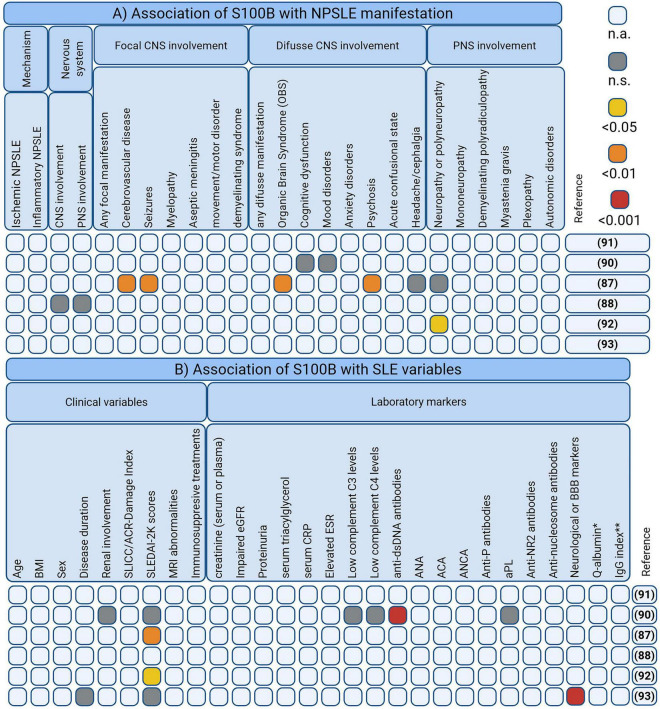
Comparative map of clinical variables related to S100B serum levels. (A) Association of S100B with NPSLE manifestation and (B) Association of S100B with SLE demographic and clinical variables. This figure provides a comparative overview of how different studies, using diverse statistical approaches, have examined the relationship between serum S100B and clinical variables. Readers can interpret the grid by following the color coding, which indicates statistical significance: Red, *p* < 0.001; Orange, *p* < 0.01; Yellow, *p* < 0.05; Gray, not significant; Blue, not assessed. In this way, the figure highlights both consistently reported associations and underexplored aspects. Further details of each association are described in the main text. NPSLE, neuropsychiatric systemic lupus erythematosus; CNS, central nervous system; PNS, peripheral nervous system; BMI, body mass index; SLICC/;ACR, systemic lupus international collaborating clinics/american college of rheumatology; SLEDAI-2K, systemic lupus erythematosus disease activity index 2000; MRI, magnetic resonance imaging; eGFR, estimated glomerular filtration rate; CRP, C-reactive protein; ESR, erythrocyte sedimentation rate; anti-dsDNA antibodies, anti-double-stranded deoxyribonucleic acid antibodies; ANA, antinuclear antibodies; ACA, anticentromere antibodies; ANCA, anti-neutrophil cytoplasmic antibodies; Anti-P, anti-ribosomal P; aPL, antiphospholipid antibodies. Q-albumin, quotient albumin*, is the ratio between CSF/Serum albumin, higher Q-albumin indicates BBB disruption. IgG index [(CSF/S IgG ratio)/(CSF/S albumin ratio)], higher IgG index indicates IgG CNS production. Created with BioRender.com.
